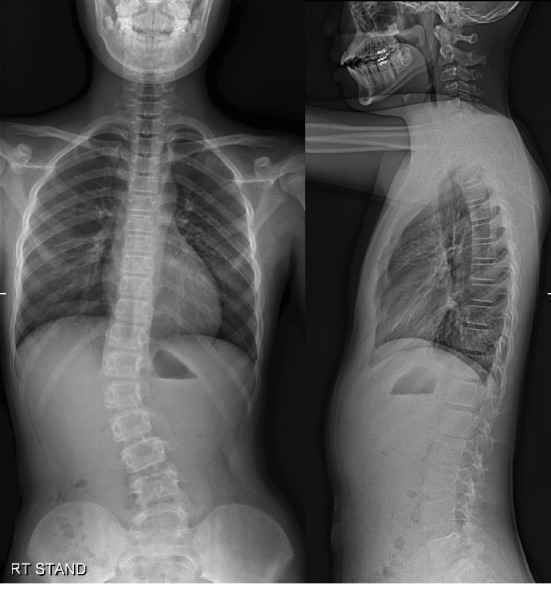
Whole spine plain radiograph. AP and lateral view when brace therapy was started, at June 16^th^, 2014.
